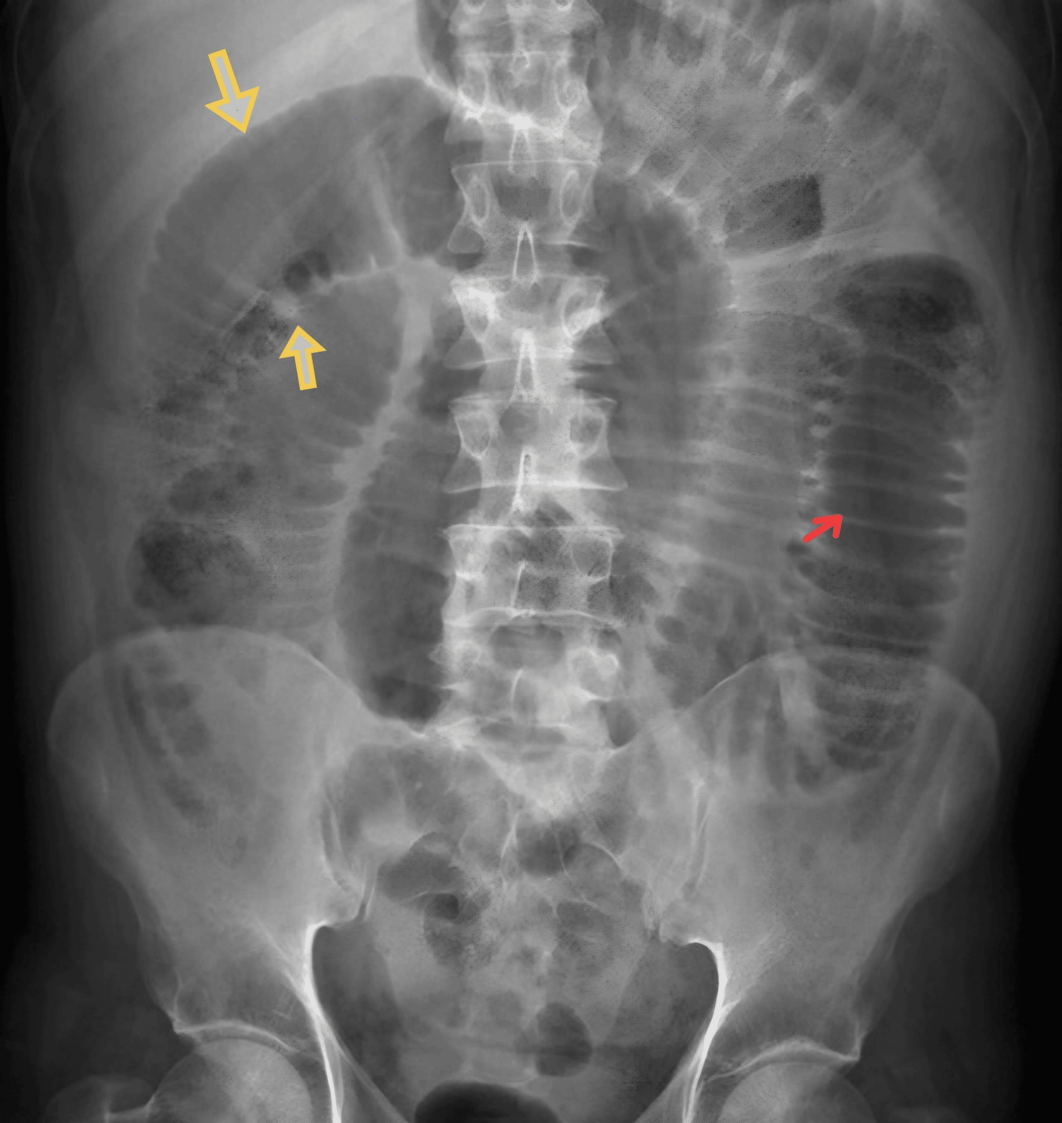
X-ray erect abdomen showing multiple dilated bowel loops suggestive of small bowel obstruction In small bowel obstruction, the centrally positioned gas-filled and distended bowel loops (marked by yellow arrows) are noted. The white lines traversing the full width of the bowel are valvulae conniventes (marked by red arrows), which are characteristic of the small bowel.
